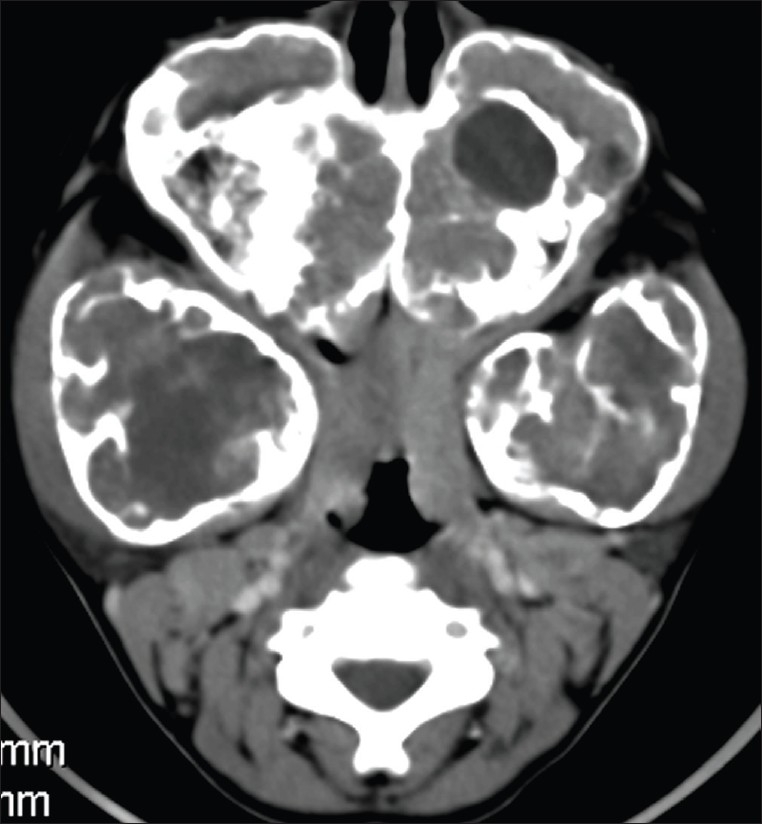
Axial CT image shows expansile lesions in the mandible and the maxilla, with mildly sclerotic matrix and internal trabeculations
